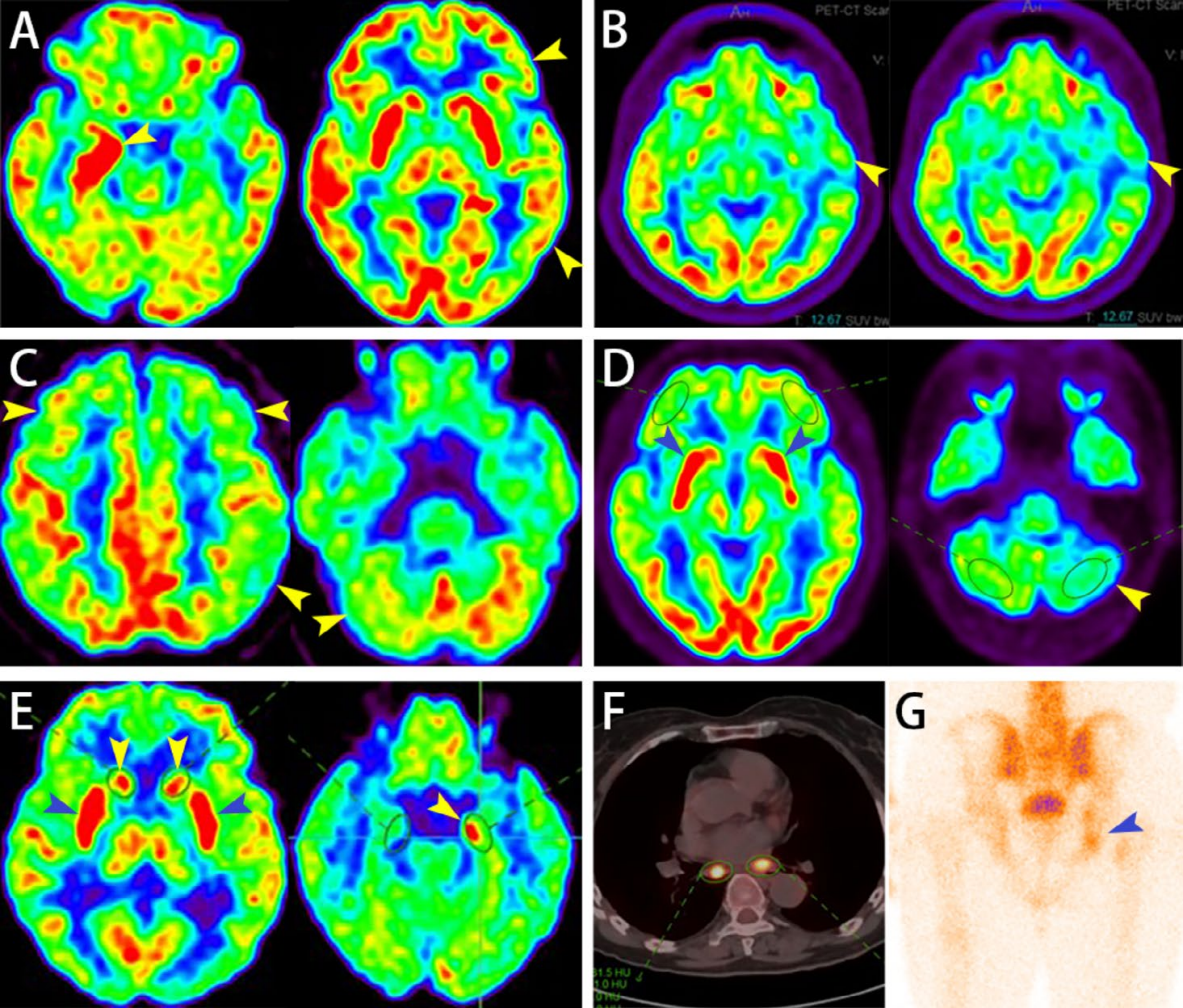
The distinctive PET‐CT/SPECT imaging features of GAD65 neurological autoimmunity. (A) A 50‐year‐old female patient with limbic encephalitis exhibits abnormal hypermetabolism in the right hippocampus and hypometabolism in the left frontal and temporal lobes. (B) A 24‐year‐old male patient with epilepsy exhibits prominent hypometabolism in the right temporal lobe cortex, corresponding with the lesion region on the brain MRI (see Figure 1B). (C) A 63‐year‐old male patient with parkinsonism demonstrates mild to severe hypometabolism in the bilateral frontal, parietal, and temporal lobes, most notably in the left frontal, parietal, and parieto‐occipital junction, with mild hypometabolism in the right cerebellar hemisphere (His brain MR imaging; see Figure 1C). (D) A 77‐year‐old male patient with parkinsonism demonstrates hypermetabolism in the bilateral caudate nuclei and moderate hypometabolism in the left cerebellum hemisphere (His brain MR imaging see Figure 1D). (E) A 62‐year‐old female patient with limbic encephalitis exhibits abnormal hypermetabolism in the bilateral basal ganglia and the left hippocampal. (F) A 69‐year‐old female patient with peripheral neuropathy revealed hypermetabolism of the multiple enlarged lymph nodes in both pulmonary hila and mediastinum. (G) SPECT revealed abnormally hypermetabolism in the right acetabulum and localized ischium of a 56‐year‐old female patient with LEMS, with tumor metastasis not excluded. LEMS, Lambert‐Eaton Myasthenic Syndrome.
